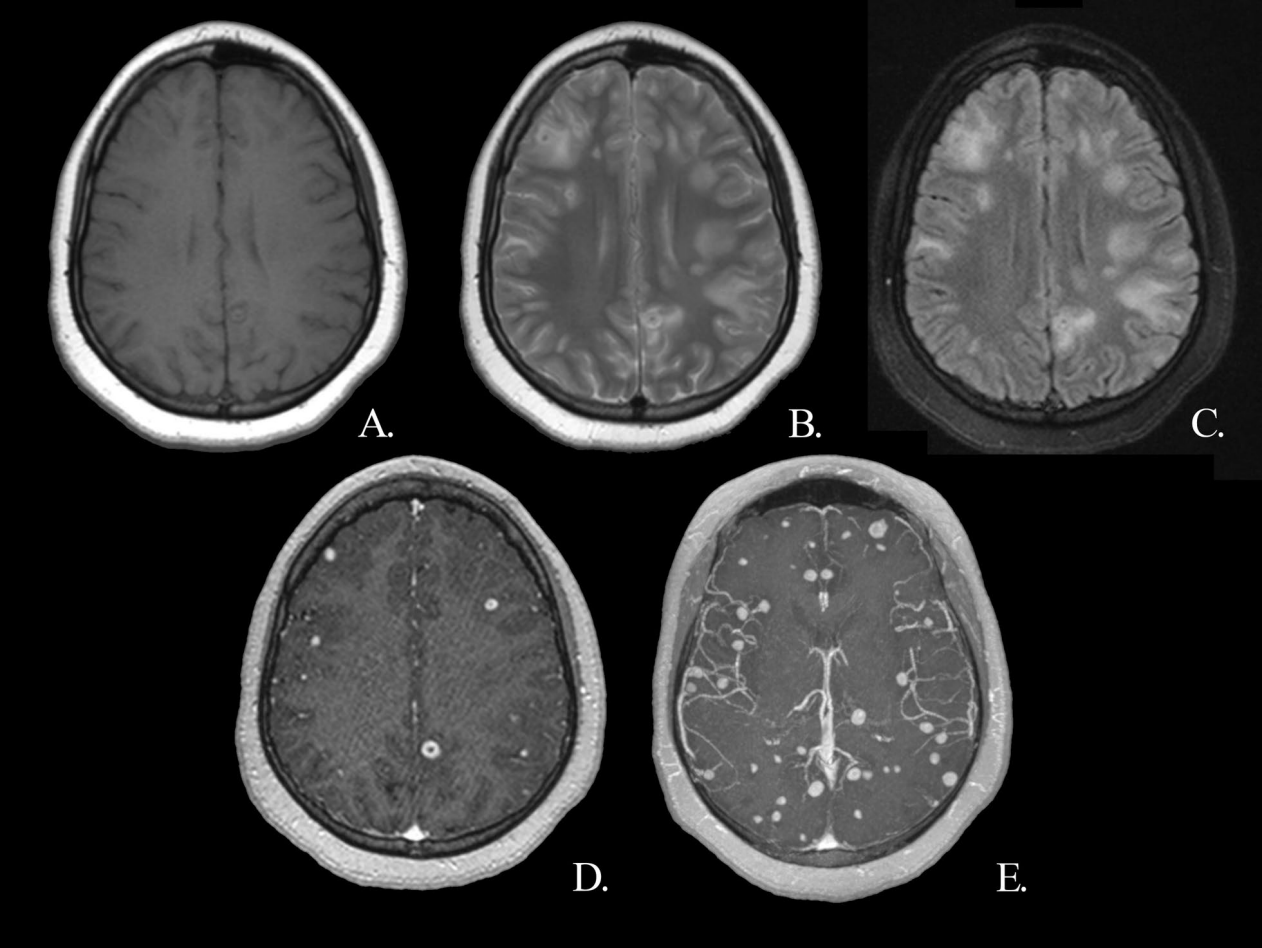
Patient's first MRI on admission. Multiple hypointense, nodular lesions ranging in size from 5 to 9 mm were observed on T1W (A), T2W (B), and FLAIR C images, showing infratentorial and supratentorial distribution with partial preservation of the central parts. The lesions did not show diffusion restriction (not shown) and there was surrounding vasogenic edema, which was observed as a patchy signal increase on T2 and FLAIR images (B, C). There was no signal loss in the suscebtibility-weighted images (not shown). In contrast-enhanced images, ring-like enhancement was observed mostly, and homogeneous nodular enhancement was observed in a few smaller lesions (D). Note the high number and watershed localization of the lesions on post-contrast T1W axial MIP images (E). *FLAIR* fluid-attenuated inversion recovery, *MIP* maximum intensity projection, *T1W* T1 weighted, *T2W* T2 weighted
